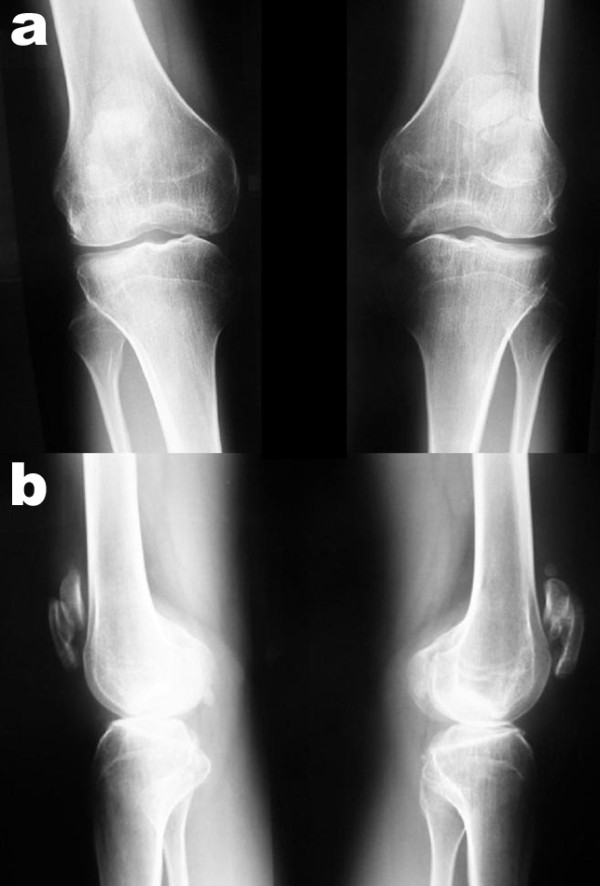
Preoperative x-rays (a: anterior posterior and b: lateral views) of both knees demonstrating multilayered patellae and dysplastic medial tibial plateaus.
